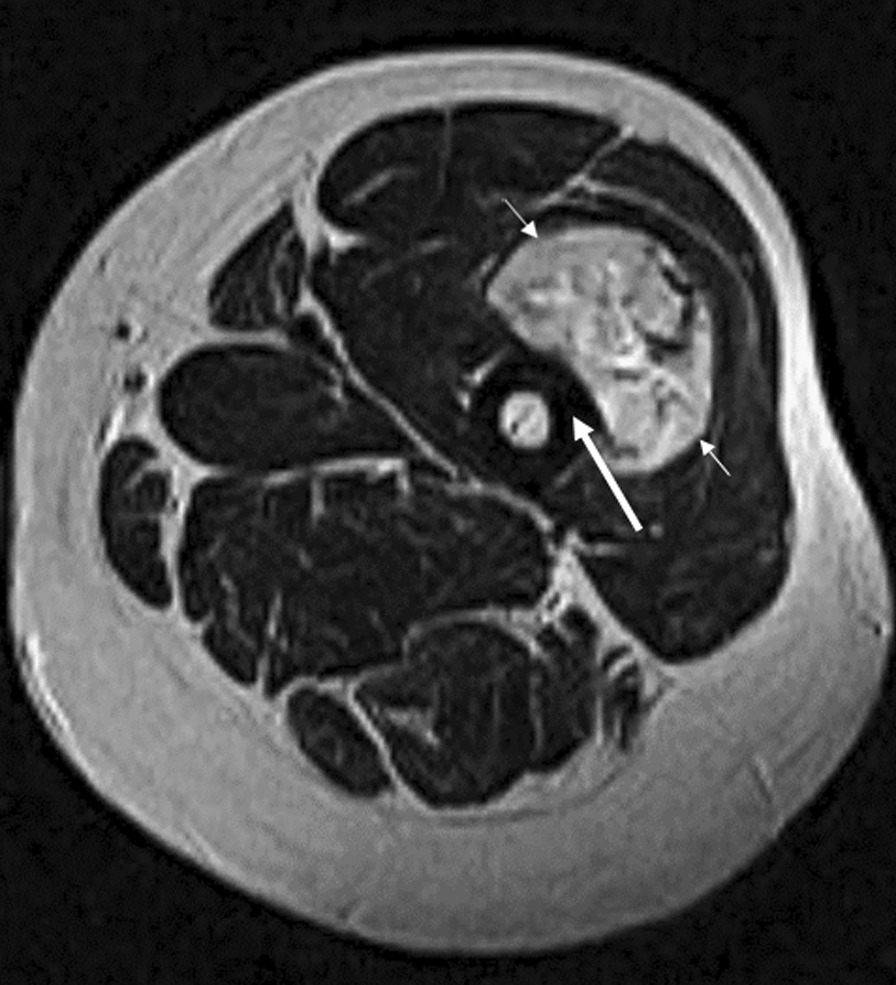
Axial T2-weighted magnetic resonance (MR) image showing a deep mass (short arrows) with high signal intensity corresponding to the primitive lesion (ASPS). The lesion is in contact with the cortical bone without edema or osteolysis (long arrow) *MR* magnetic resonance, *ASPS* alveolar soft part sarcoma
